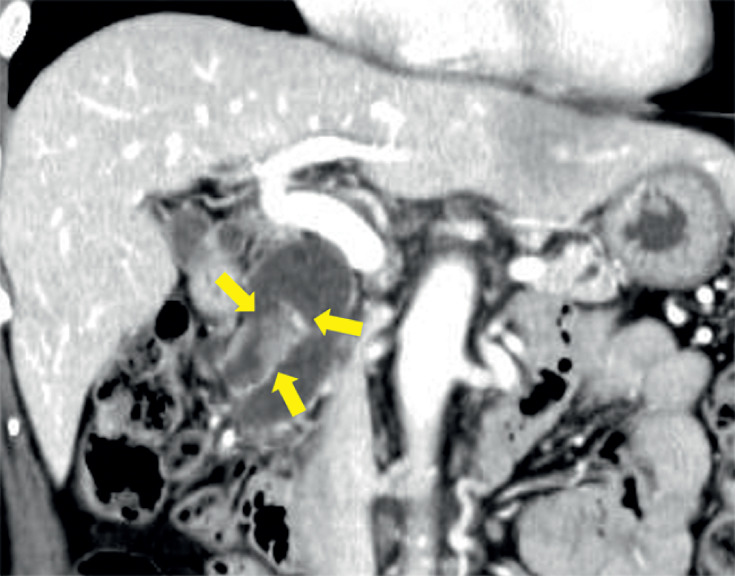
Contrast-enhanced computed tomography revealed a 10-mm tumor in the accessory pancreatic duct.
